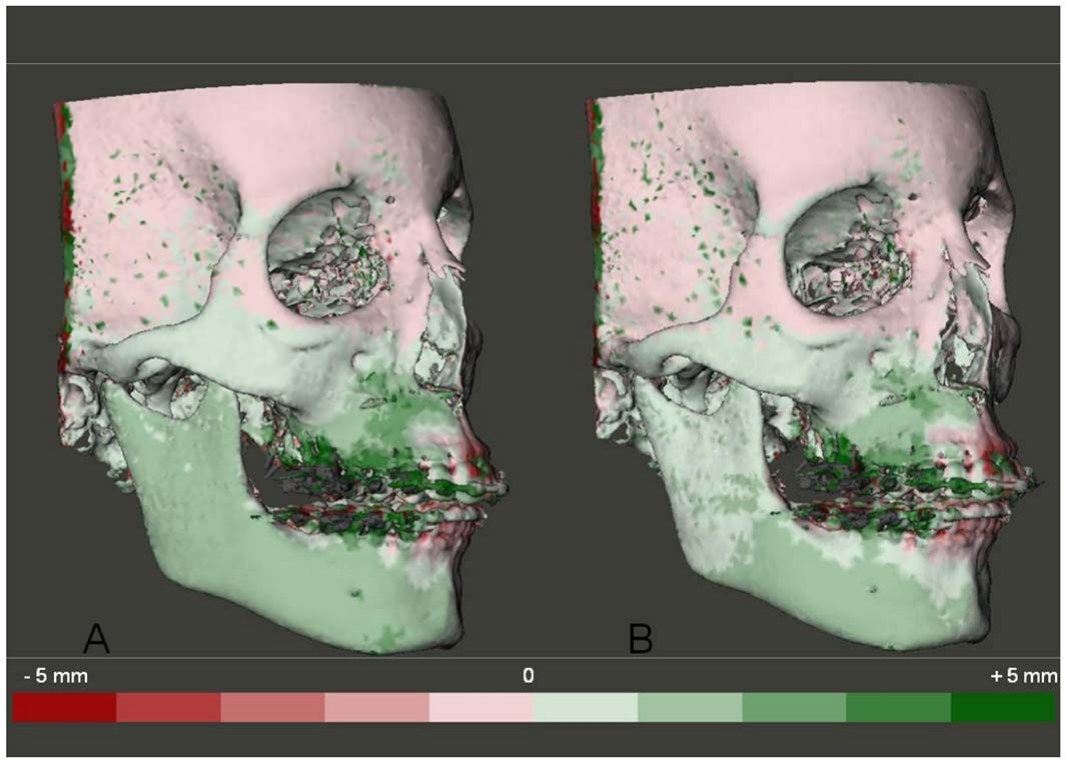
Color coded distance maps to visualize treatment changes following two CBCT scans superimposition. The green color indicates that the superimposed model is in front of the original model and red color indicates the opposite. Each color graduation is 1 mm. (A) models registered on the anterior cranial base. (B) same models registered on the left zygomatic arch.
